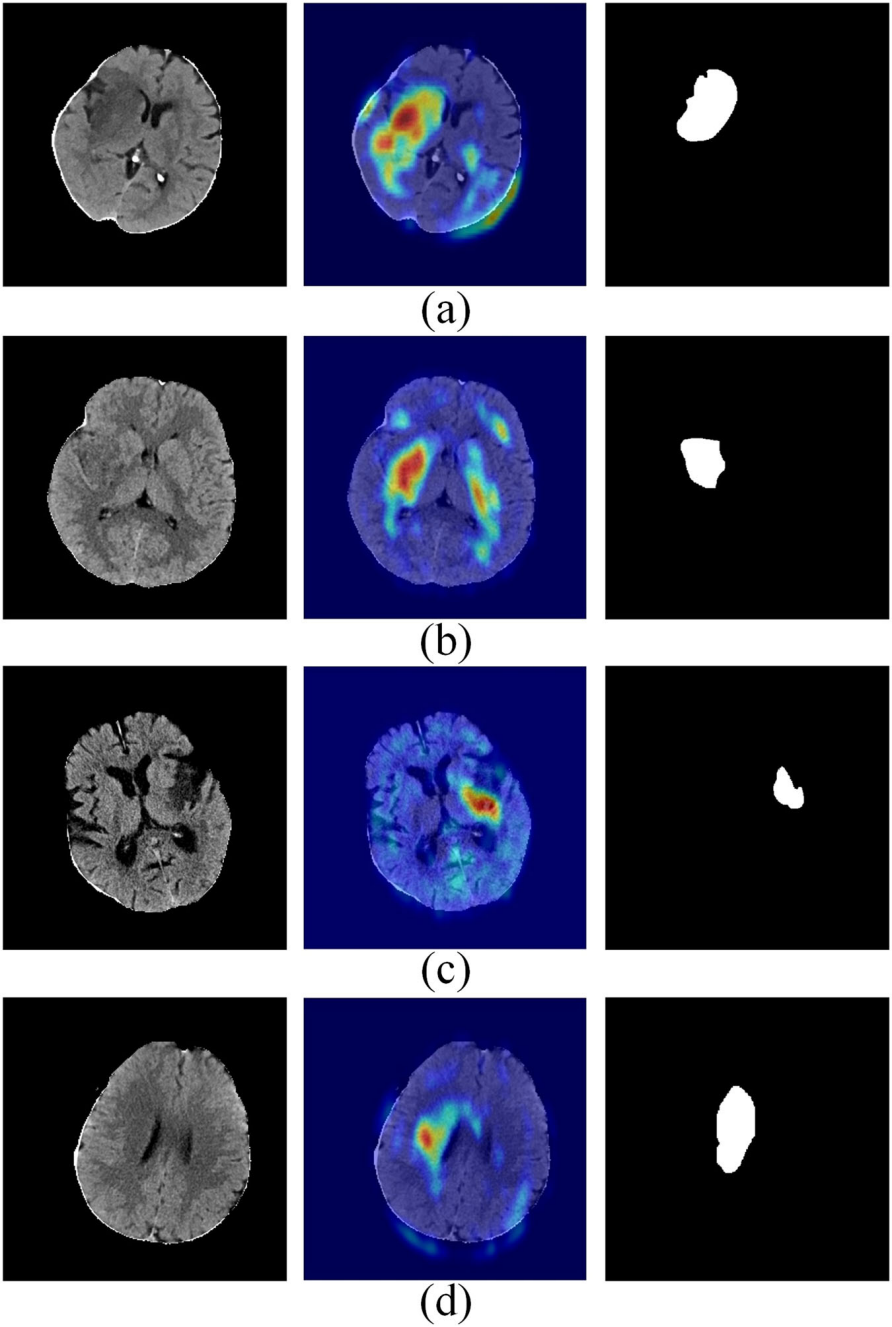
Visualization of the imaging model for hemorrhagic transformation prediction using the Grad-CAM++ method. A 74-year-old man (6 h from onset to CT scan) (a) and A 66-year-old woman (3.5 h from onset to CT scan) (b) were confirmed to have undergone hemorrhagic transformation based on follow-up images. A 78-year-old man (9 h from onset to CT scan) (c) and a 58-year-old woman (3 h from onset to CT scan) (d) were confirmed to have had a stroke without hemorrhagic transformation. The first column shows the original image, the second shows the mask of the lesion, and the third shows the class activation map. Darker red indicates a greater contribution for hemorrhagic transformation prediction, while darker blue indicates a lower contribution in the class activation map
